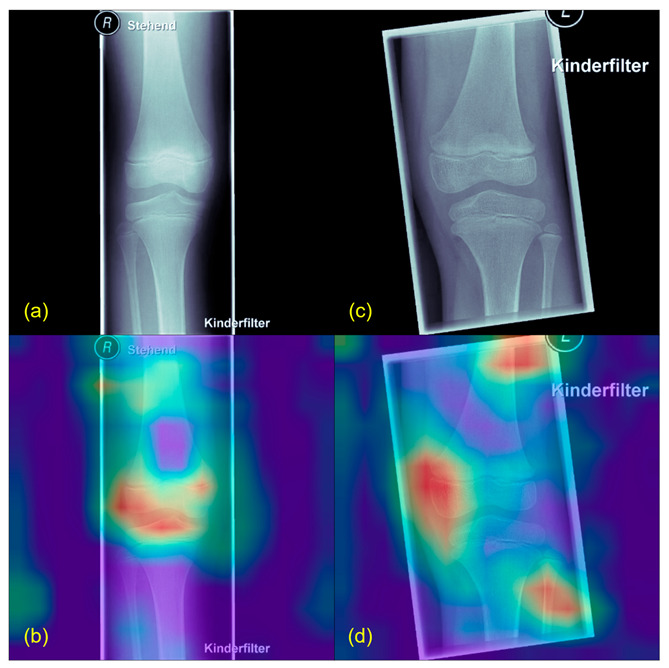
Grad-CAM example from healthy patients. (a,c) show the original X-rays, (b,d) the Grad-CAMs of the same images. Highlighted in red are the parts used by the algorithm to find its conclusion. Patients classified as healthy usually showed a more diffuse Grad-CAM.
